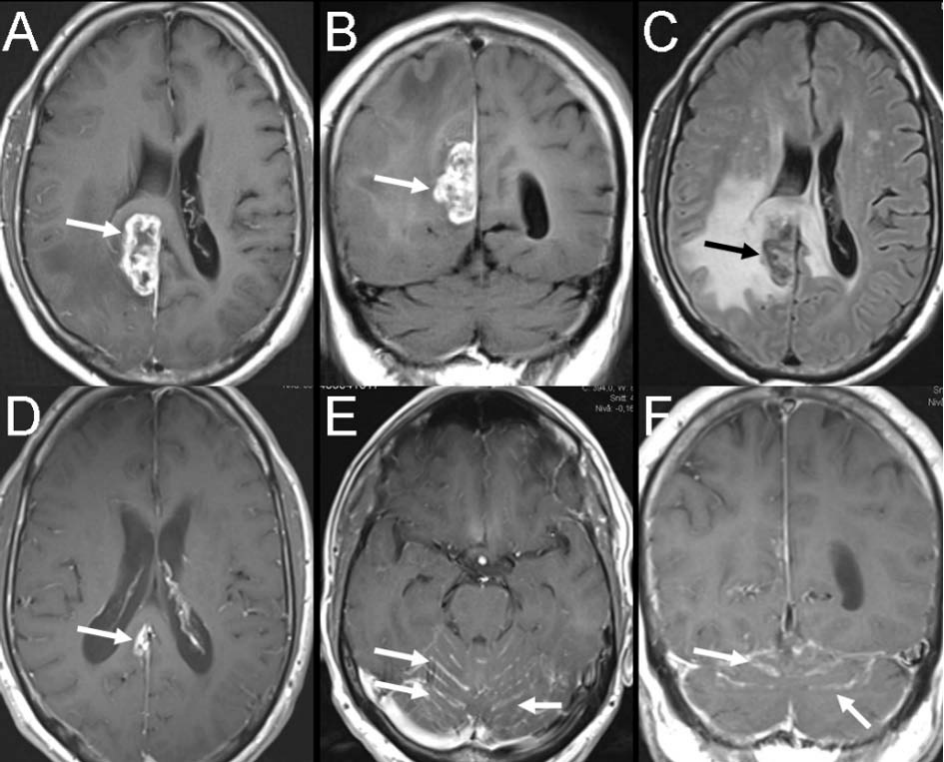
Axial magnetic resonance imaging (MRI) scan at three different occasions. Images (A-C) show the initial MRI with a large right-sided supratentorial meningeal tumor (arrows) with extensive surrounding edema (bright signal, C). D) MRI after treatment with gamma knife shows marked reduction of the tumor size with only little residual tumor (arrow). E-F) MRI six months from the start of symptoms shows leptomeningeal metastases with linear contrast enhancement along the cerebellar sulci (arrows).
